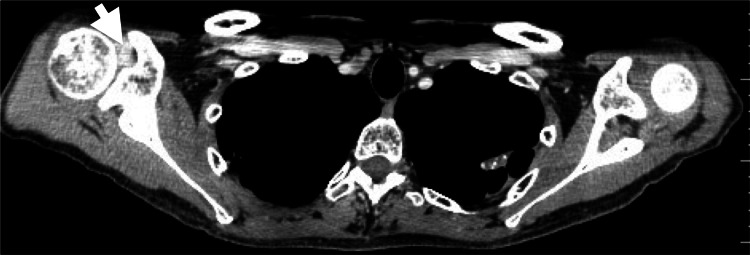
Case 1. Axial CT of CK-SBRT planning showing a contrast-enhanced lesion at the rotator interval of the right shoulder joint. CT: computed tomography; CK-SBRT: stereotactic body radiotherapy using CyberKnife
